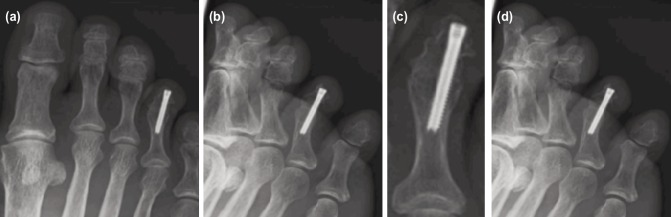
Post-operative radiographs at six months, showing union of right fourth toe distal phalanx fracture and inter-phalangeal fusion. (a) Dorso-plantar foot view, (b) Oblique foot view, (c) Magnified dorso-plantar fourth toe view, (d) Magnified oblique fourth toe view.
